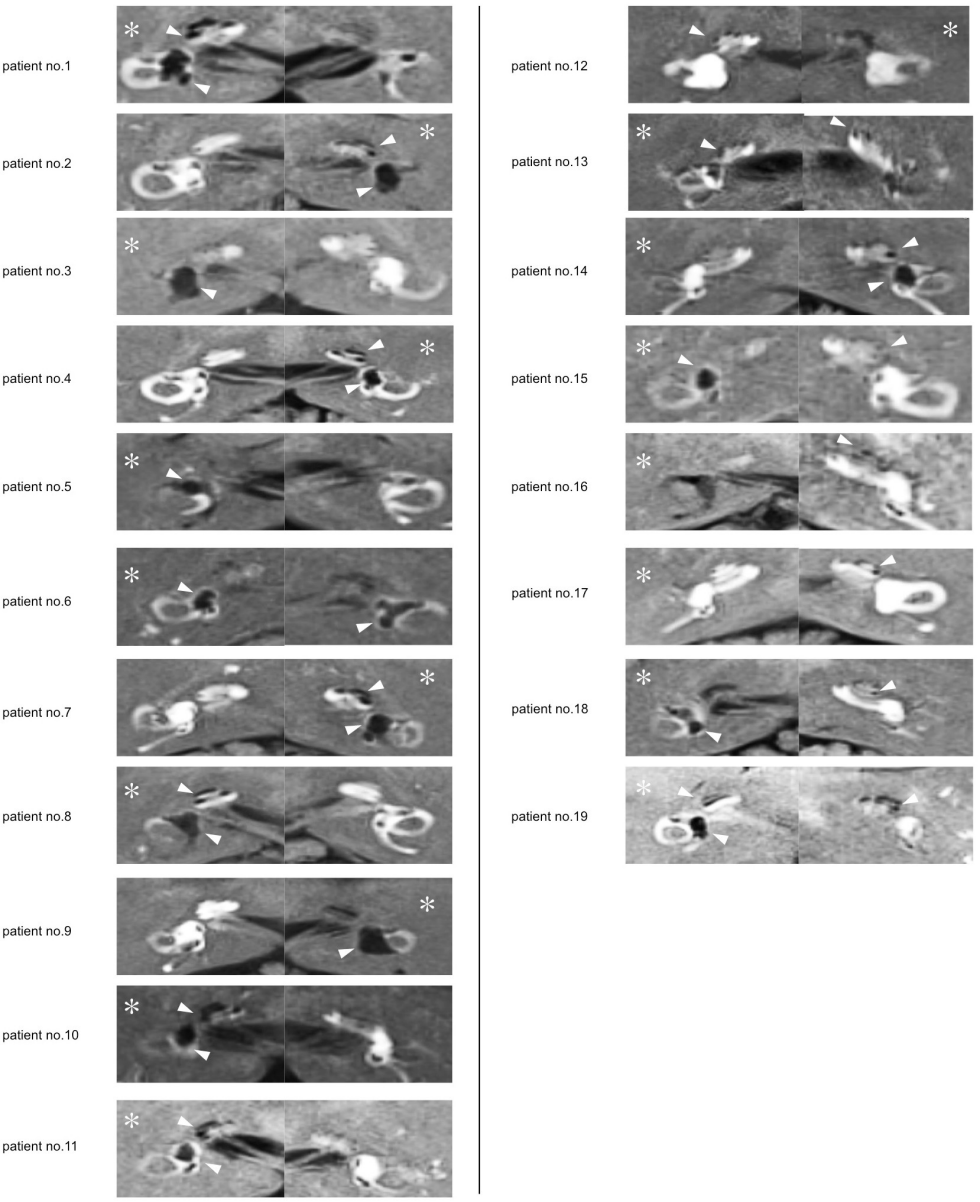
MR images of all patients participating in this study. The perilymph space was filled with gadodiamide and enhanced as a white area. The endolymphatic hydrops (ELH) is detectable as a black area (arrow head). Ipsilateral DEH are shown in the left column and contralateral DEH in the right column. The side of the precedent hearing loss ear is marked with an asterisk.
